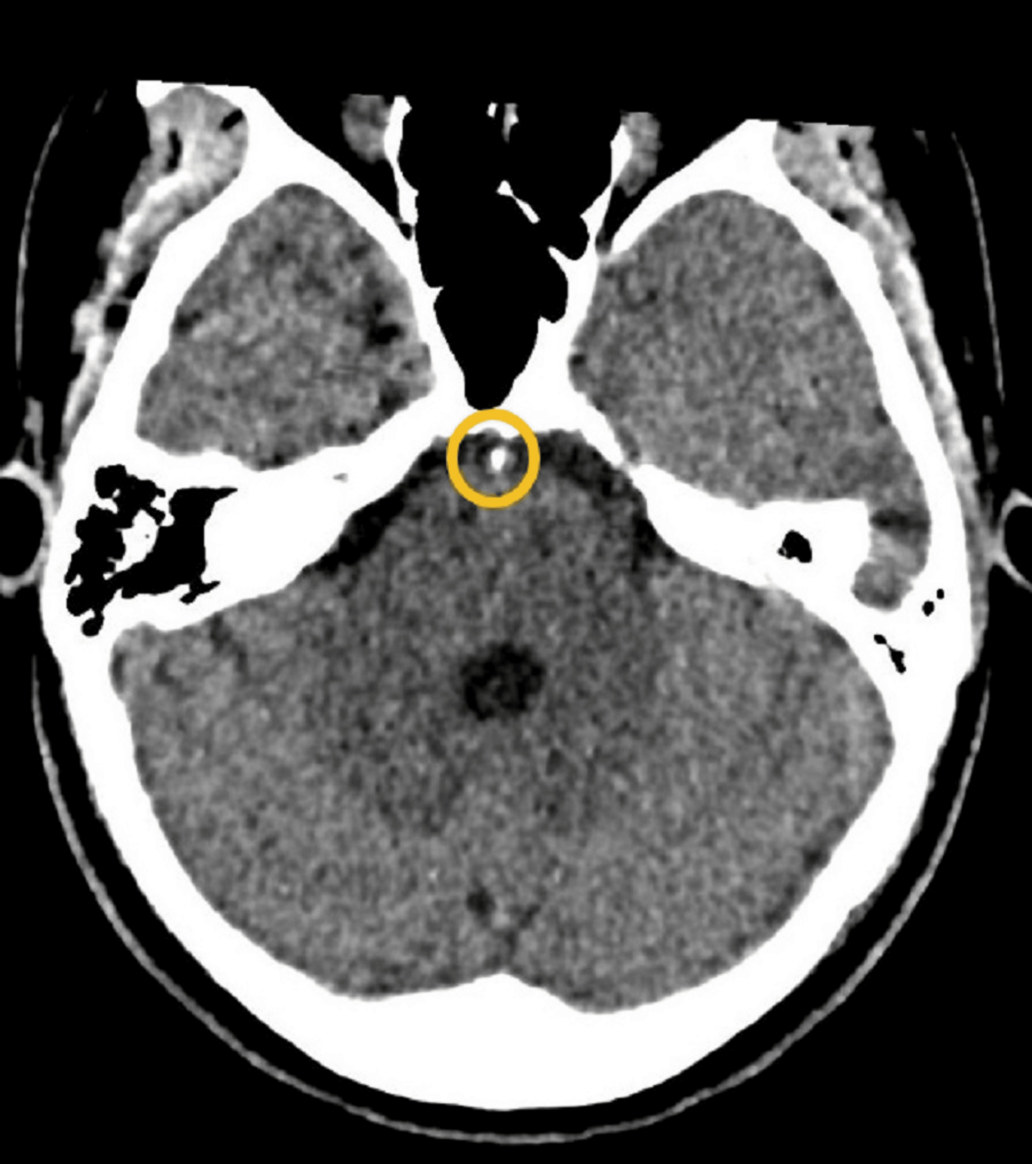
CT head, unenhanced: Axial image showing a focal hyperdensity (mean Hounsfield unit = 150) at the level of the mid-basilar artery, thought to represent an atherosclerotic plaque/calcification or possibly a small thrombus.
